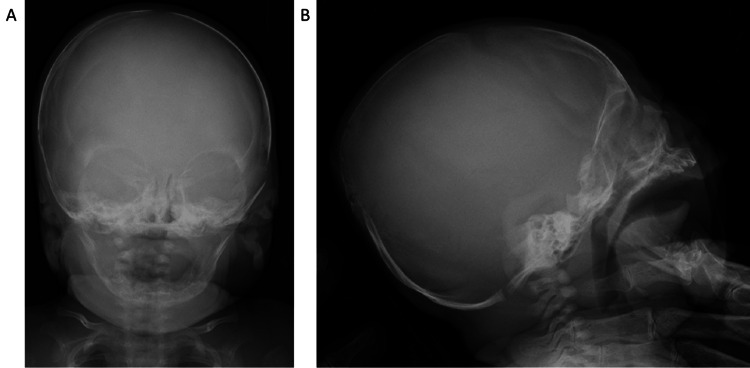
Radiograph of skull at two months of age. (A) Anteroposterior view and (B) lateral view. There is no evidence of Wormian bones.
